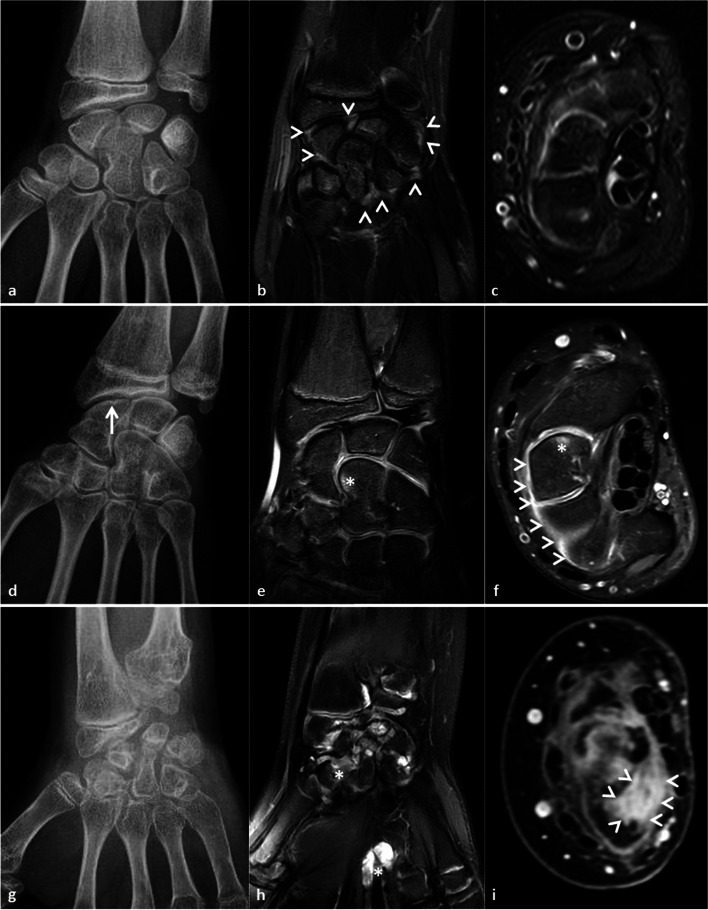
Radiological findings of Dry Synovitis in comparison with RF negative polyarticular JIA. (a- > c) Imaging of the right wrist of an 11y old girl with DS. a Plain X-ray: no significant bone erosion. b Fat suppressed T1-weighted gadolinium-enhanced MRI, frontal view: diffuse mild synovial hypertrophy (arrow heads) with moderate gadolinium enhancement, involving joint synovium (with similar findings on peritendinous synovium). c Fat suppressed T2-weighted MRI, axial view: no significant joint or peritendinous effusion. (d- > f) Imaging of the right wrist of a 13y old girl with DS d. Plain X-Ray: subtle carpal bone erosion (arrow). e Fat suppressed T2-weighted MRI, frontal view: bone marrow oedema (*) with no joint effusion. f. Fat suppressed T1-weighted gadolinium-enhanced MRI, axial view: diffuse synovial joint enhancement (arrow heads) and bone marrow oedema (*). (g- > i) Imaging of the right wrist of a 11y old girl with polyarticular JIA. g Plain X-Ray: diffuse severe bone erosion. h Fat suppressed T2-weighted MRI, frontal view: joint effusion involving both joint and peritendinous component (*). i Fat suppressed T1-weighted gadolinium-enhanced MRI, axial view: diffuse proliferative hypertrophy of joint synovium (arrow heads) with intense gadolinium enhancement
